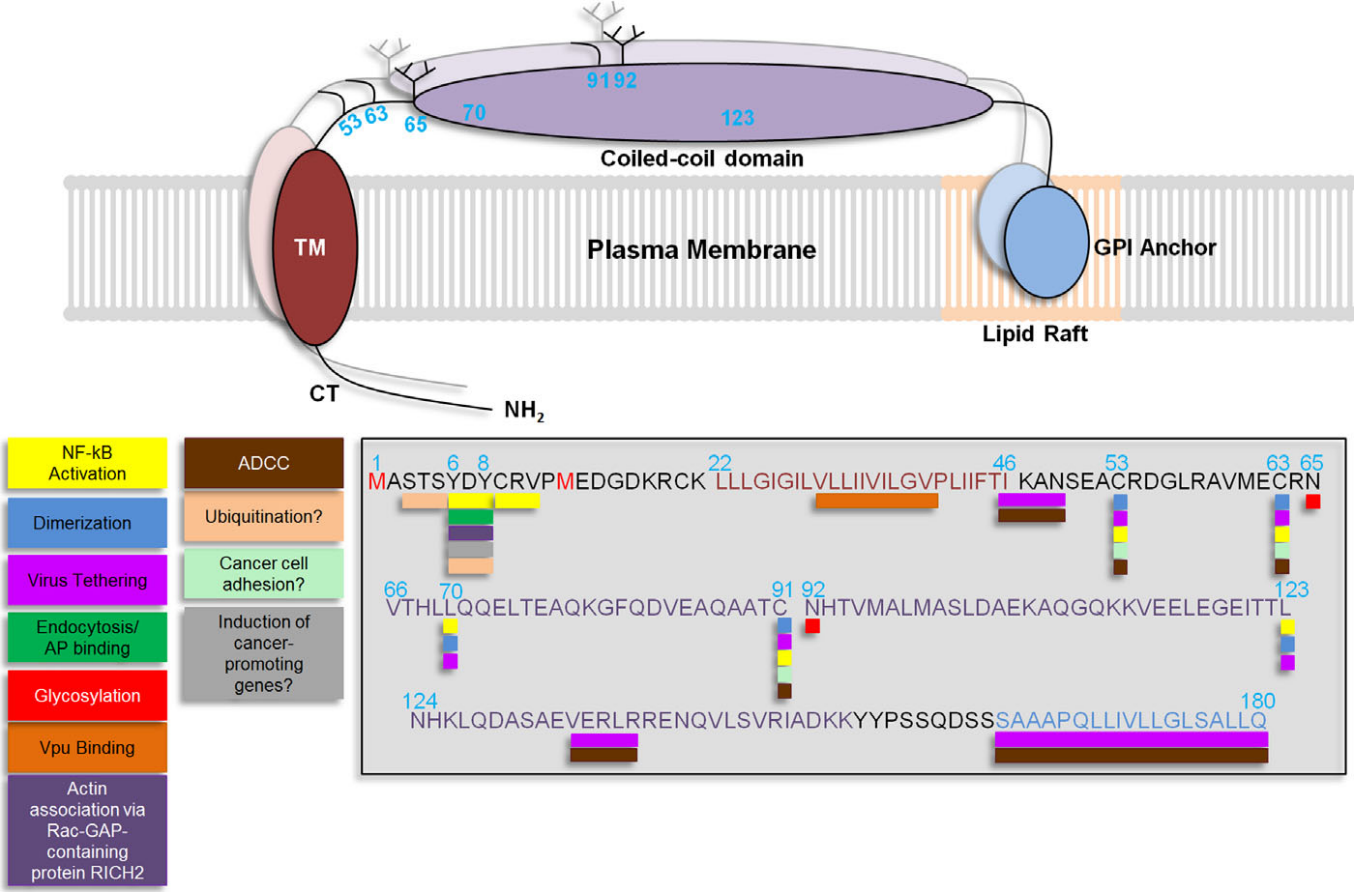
BST‐2 structure: BST‐2 is a type II transmembrane protein with a N‐terminal cytoplasmic tail (CT) followed by a transmembrane domain (TM), a coiled‐coil domain and a glycosylphosphatidylinositol (GPI) anchor embedded in lipid rafts in the cell membrane. The amino acid sequence of BST‐2 depicted in the gray box is color coded with their respective domains. Numbers on top of amino acids correspond to amino acid location. Underneath the amino acid sequences are colored boxes corresponding to different functions and characteristics of BST‐2 including NF‐κB activation (yellow), dimerization (blue), virus tethering (light purple), endocytosis/adaptor protein (AP) binding (green), sites of glycosylation (red), Vpu binding (orange), actin association (purple), motifs for ADCC induction (brown). Also included are some hypothetical characteristics/functions including sites of ubiquitination (light orange), cancer cell adhesion (light green), and induction of cancer‐promoting genes, such as matrix metalloproteinases, CXCR4, CXCL12, and other signaling molecules (gray). BST‐2 contains two translational start sites at methionine 1 and 13 (red) generating a long and short isoform, respectively. The short isoform cannot induce NF‐κB activation since it lacks the YXY motif. BST‐2 forms homo‐dimers and—tetramers through 3 conserved cytosine residues at positions 53, 63 and 91. Leucine residues at positions 70 and 123 are important for maintaining the structure of BST‐2 and for virus tethering, which also requires the C‐terminal GPI anchor.
